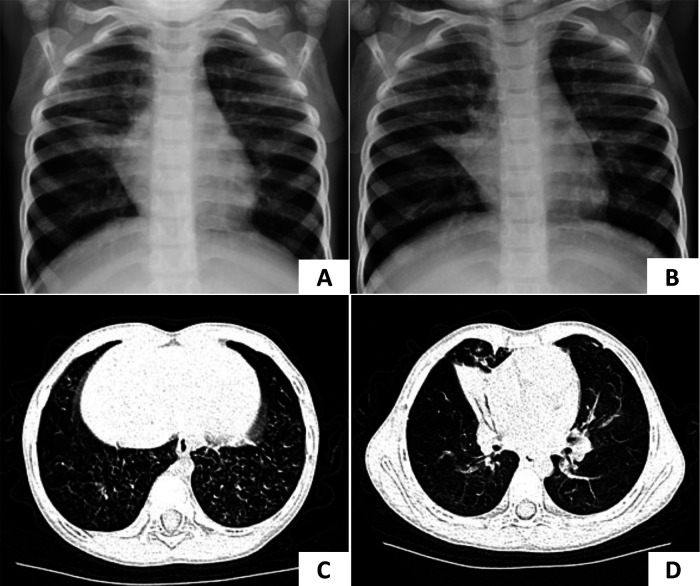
Chest radiography of case 3. (A,B) Atelectasis of the right middle lobe was persistent in two separate x-rays. (C,D) The lung CT scan showed diffuse micronodules in the lower fields of both lungs and decreased volume of the right middle lobe which were considered pneumonia with atelectasis of the right middle lobe.
